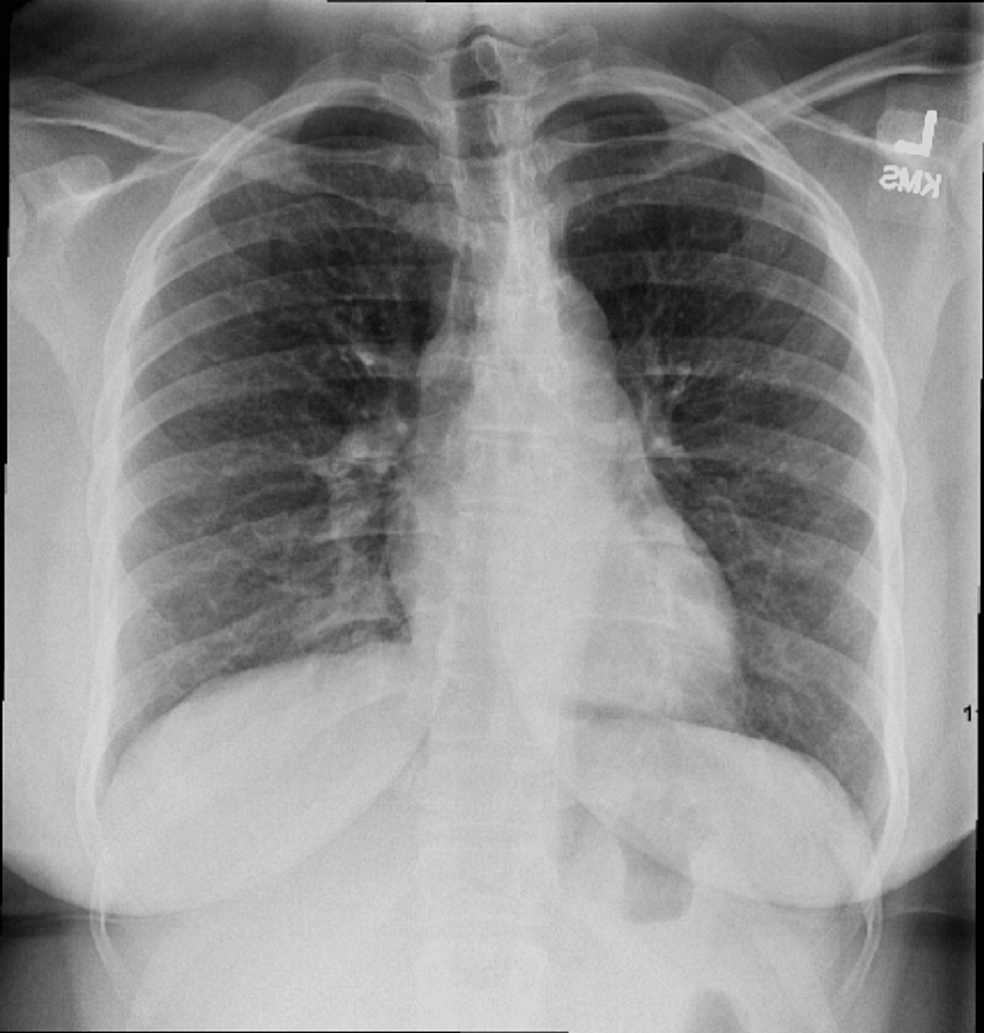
Chest x-ray on initial presentation, showing no infiltrate or any other significant changes
